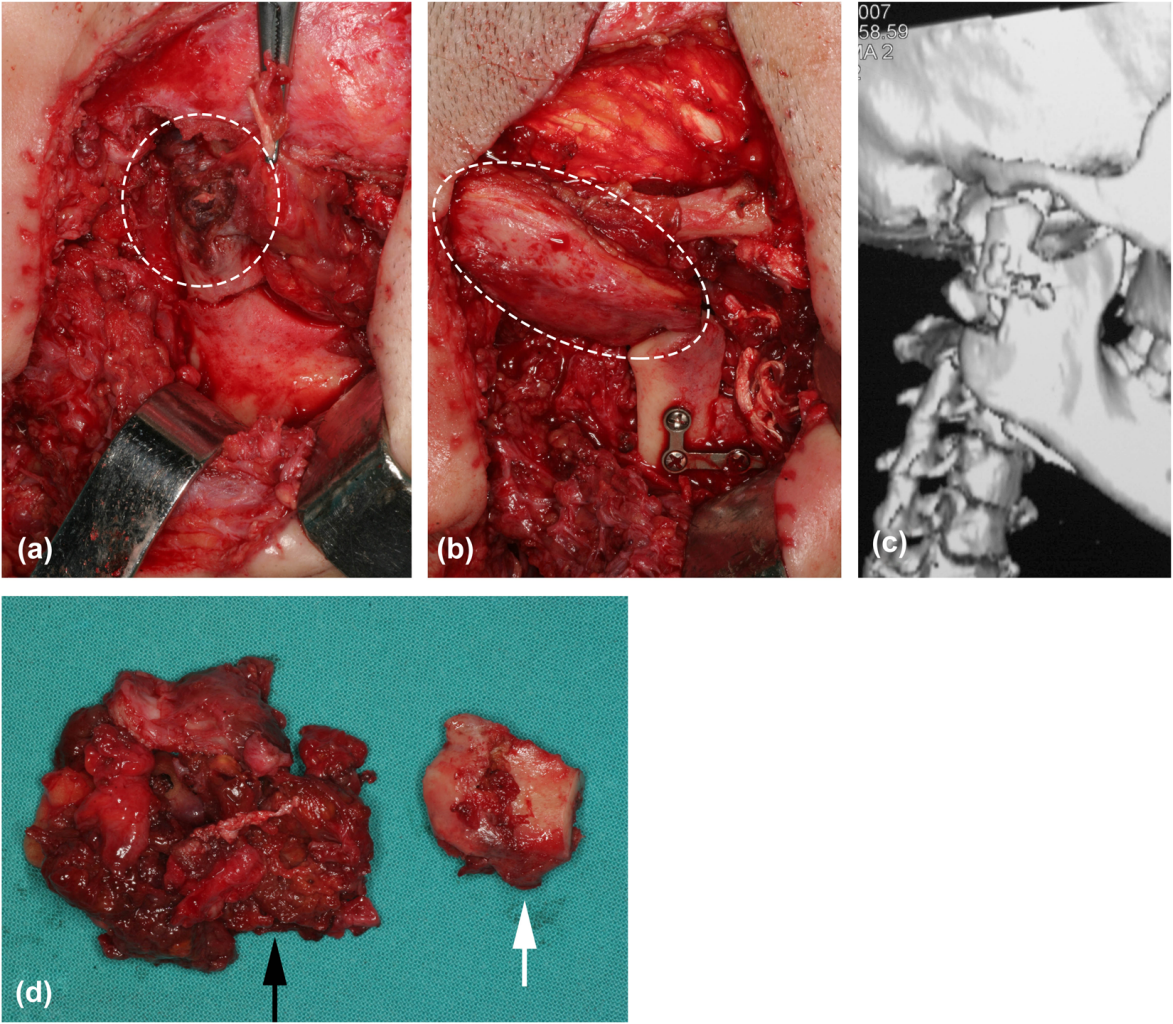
The mandibular condyle and disc were surrounded and being eroded by the neoplasm (a, white dashed region). A reverse L-shaped osteotomy line was drawn at the posterior part of the ramus. The proximal bone segment was then moved superiorly to reconstruct the mandibular condyle and fixed using an L-shaped miniplate (b, white dashed region shows temporal muscle). (c) A 3D reconstruction of postoperative CT images show the reconstructed mandibular condyle. (d) Depicts the neoplasm (black arrow) and damaged mandibular condyle (white arrow).
